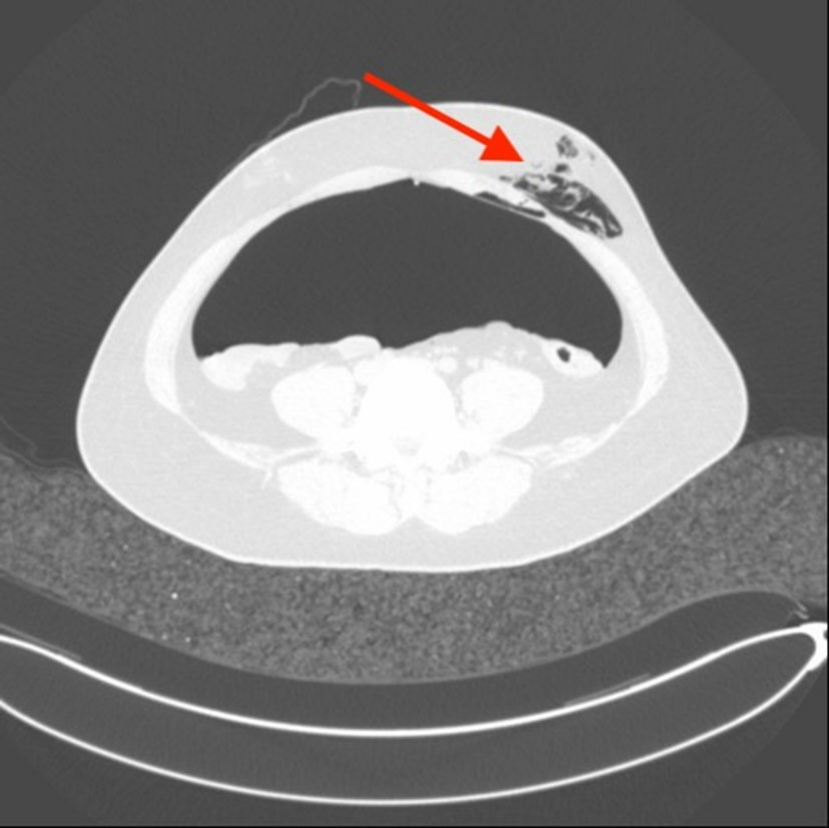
Complications of CT pneumoperitoneum. The arrow shows subcutaneous emphysema in the patient.
